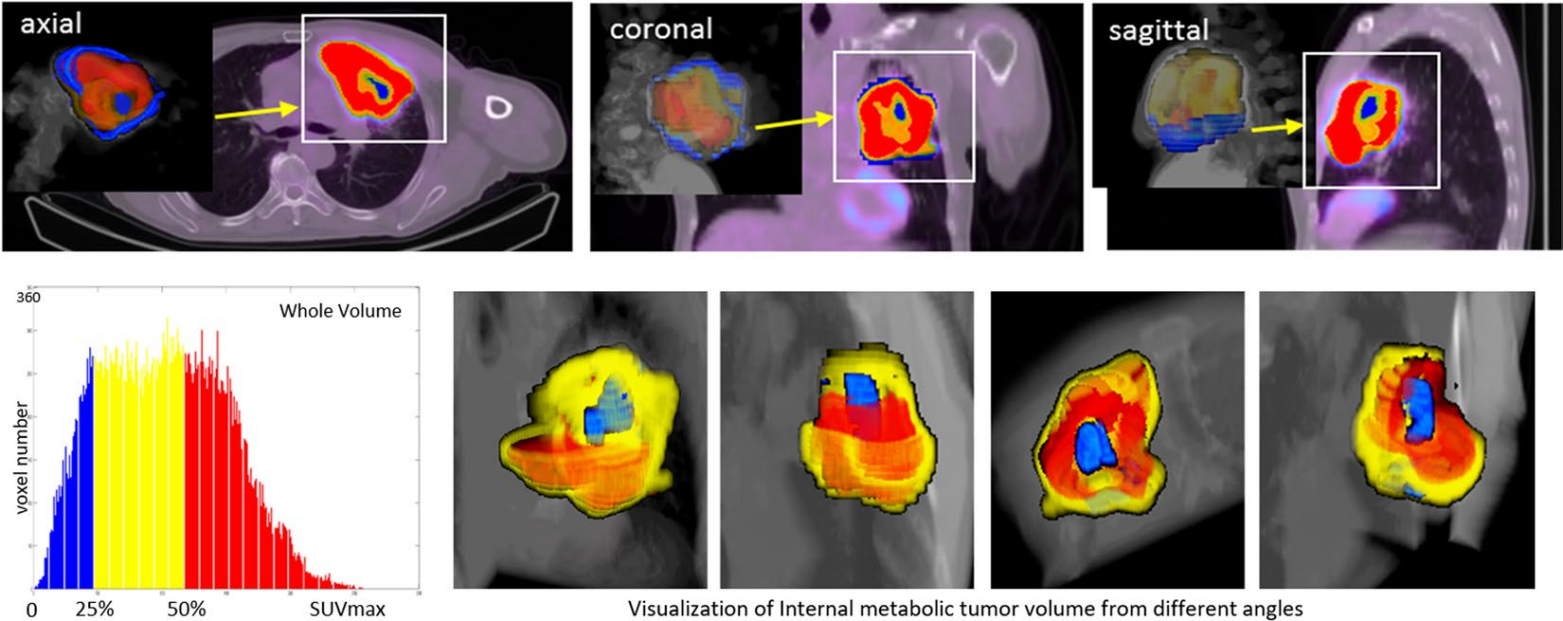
The extraction and measurement of metabolic sub-volumes and spatial SUV distributions of the case in Fig. 2(b). PTV_*high*_, PTV_*mod*_ and PTV_*low*_ are shown by red, yellow and blue colors respectively.
